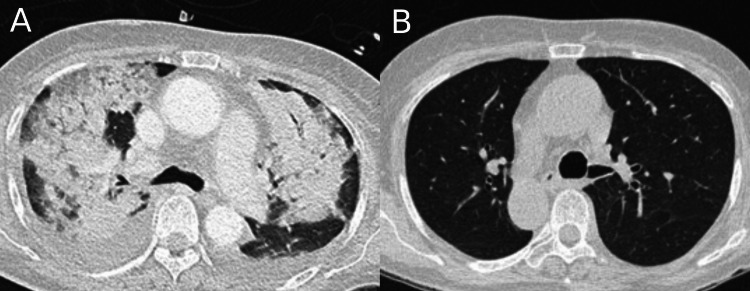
CT chest on day seven post admission (A) and three weeks after treatment initiation (B) (A) CT chest on day seven demonstrates extensive bilateral consolidation and pleural effusions, consistent with pulmonary involvement in EGPA. (B) Follow-up CT chest three weeks after initiation of immunosuppressive therapy shows marked improvement, with resolution of consolidation and significant reduction in pleural effusions.
